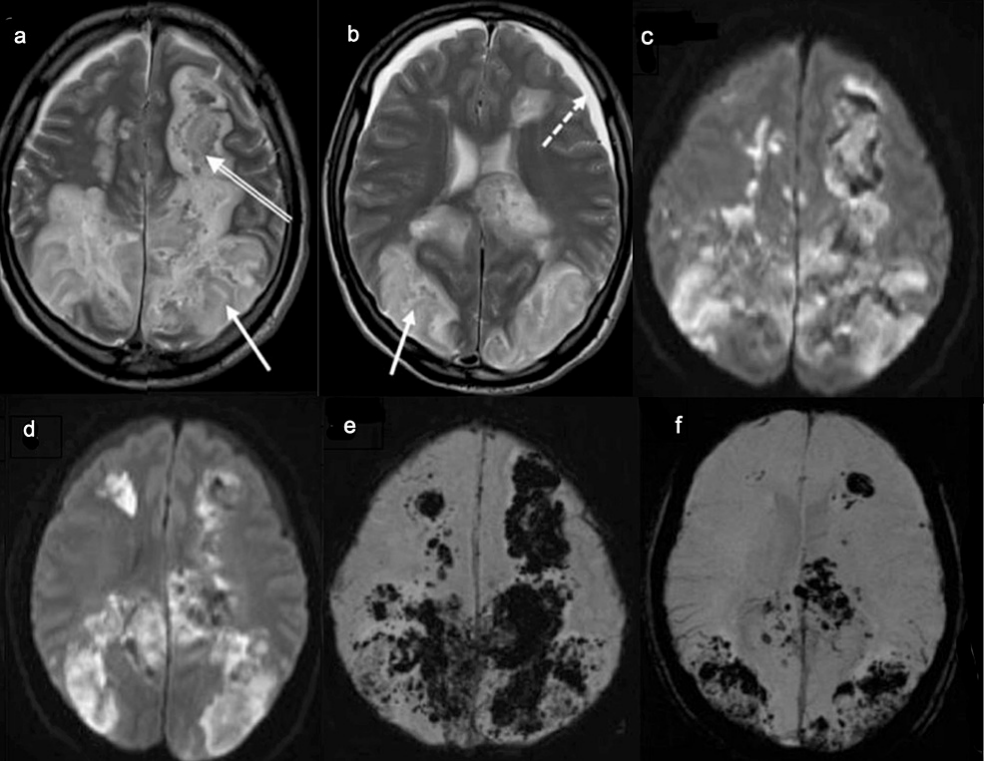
MRI of the Brain. Symmetrical bilateral white matter high T2-signal changes (a, b) with restricted diffusion on DWI (c, d) and magnetic susceptibility artifacts on SWI (e, f) in keeping with vasogenic edema with hemorrhagic changes, involving the parieto-occipital lobes (arrows), bifrontal regions “superior frontal sulcus pattern”(double arrows) and bilateral subdural effusion (dashed arrows). Features are suggestive of posterior reversible encephalopathy syndrome (PRES) complicated by lobar hemorrhage. These features were not seen in the prior exam (not shown). DWI - diffusion-weighted imaging, SWI - Susceptibility-weighted imaging
